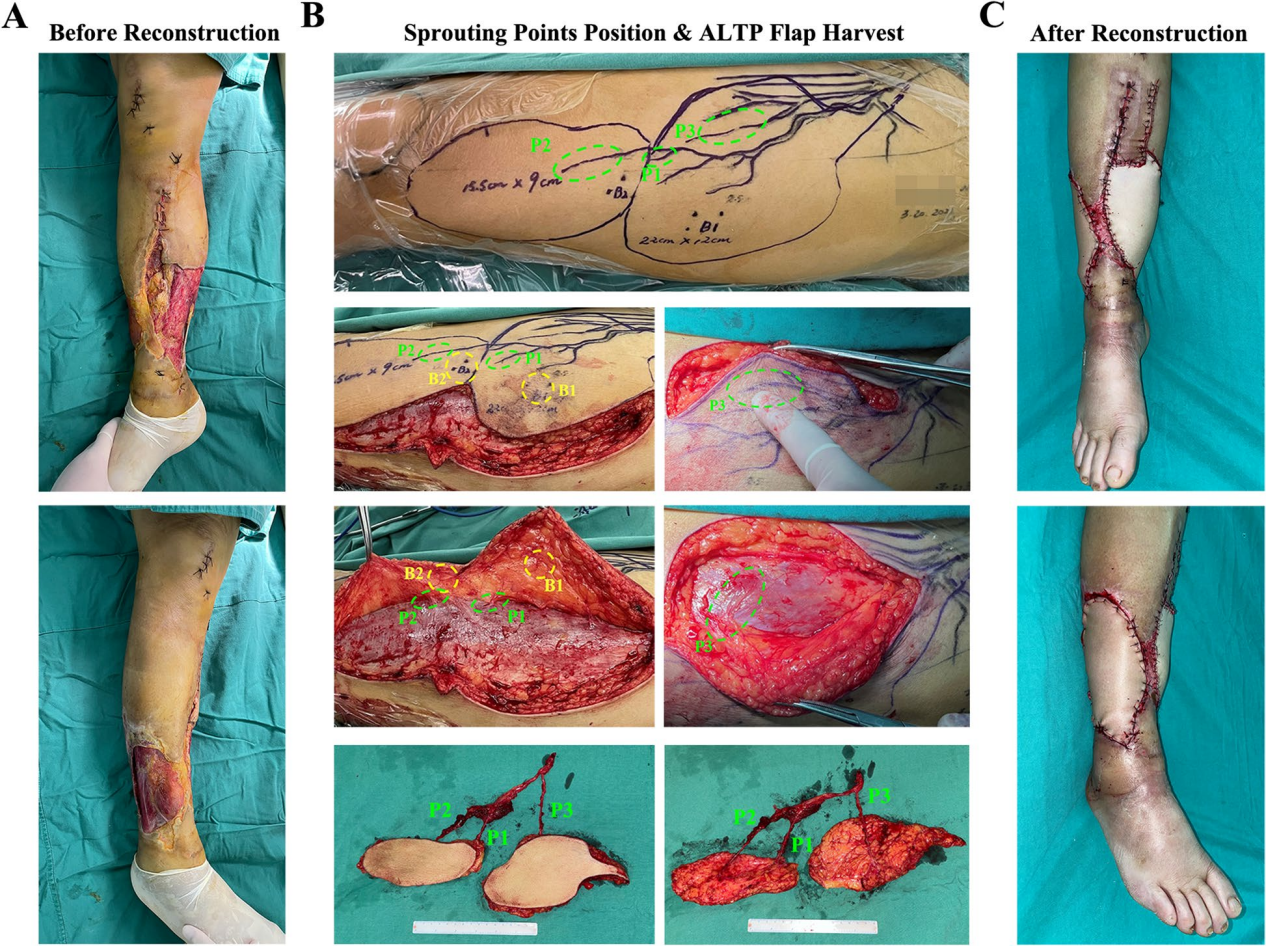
A typical case of a 52-year male. A After debridement, large soft tissue defects on the medial and lateral sides of the right calf existed, accompanied by tendon and bone exposure. B The location of the emerging point (P1-P3) came out from the deep fascia and the real-time perforators mapping were depicted on the anterolateral skin of the left thigh according to the prepared CTA images. Meantime, the pulse point of perforators (B1-B2) was also marked on the skin surface using handheld Doppler ultrasound. During operation, the lateral edge of the flap was cut up, and the cutaneous branches around the emerging points were carefully separated. It should be noticed that the emerging points of the perforators were in accordance with the preoperative marked locations determined by AR, while the points located by ultrasound were far away from the perforators. Finally, a double-foliate ALTP flap containing three perforators was obtained. C The damaged calf was reconstructed by the ALTP flap
